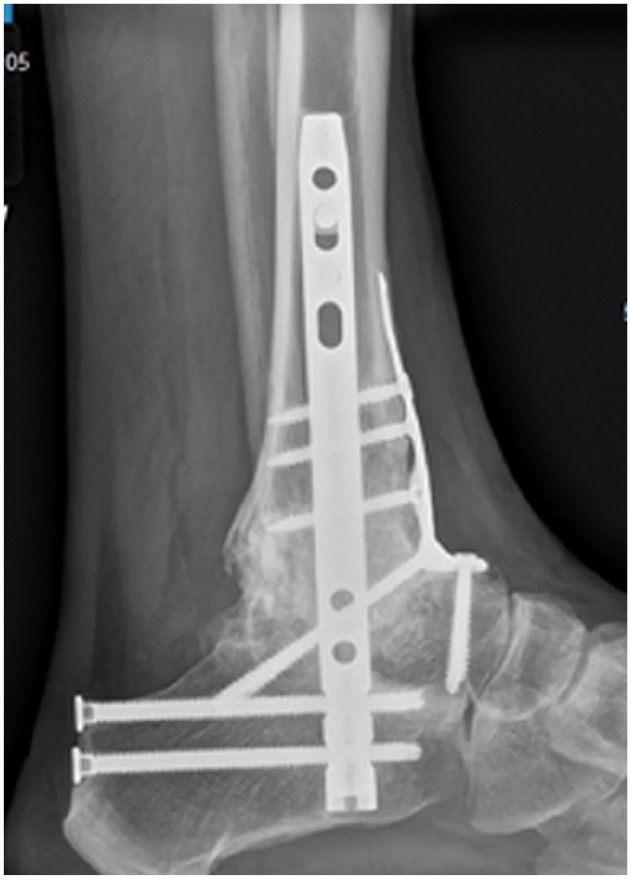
Postoperative lateral radiograph of the case in Figure 6 demonstrating tibiotalocalcaneal (TTC) fusion with intramedullary nail and anterior fusion plate using a combined anterior ankle and sinus tarsi approach. Radiographs confirm solid graft incorporation with no loss of height.
